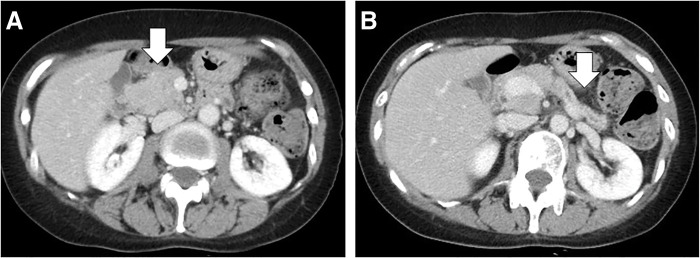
CT images revealing (A) a low-density mass measuring 42 × 30 mm in the pancreatic head (arrow) and (B) dilatation of the main pancreatic duct (arrow).
